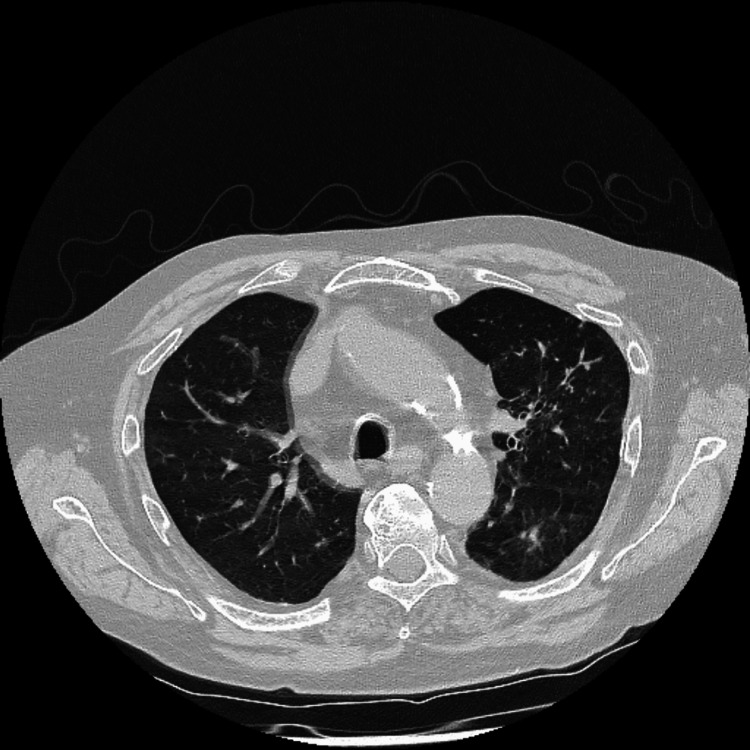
High-resolution CT scan of the lungs showing improving picture compared to previous scans CT: computed tomography
